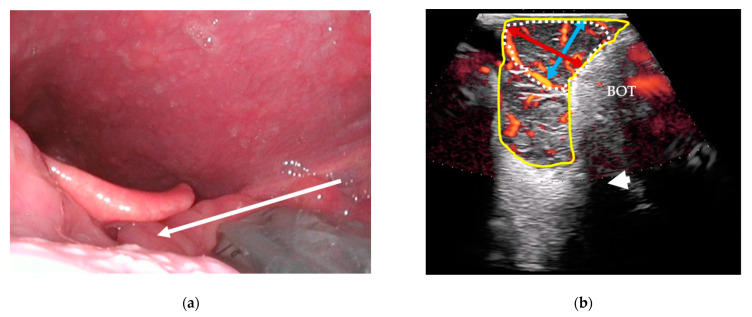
Transoral US of the lingual tonsils: (a) a sheathed US transducer placed on the left lingual tonsil (arrow: swipe direction lateral-to-midline) in the sagittal plane; (b) sagittal US image with Power Doppler of the lingual tonsil (yellow outline), a tumor-suspected area (dotted outline), underlying base of tongue musculature (BOT), and the hyoid bone’s acoustic shadow (white arrowhead). The craniocaudal (blue bi-directional arrow) and anteroposterior (red bi-directional arrow) diameters are shown on the tumor-suspected area.
